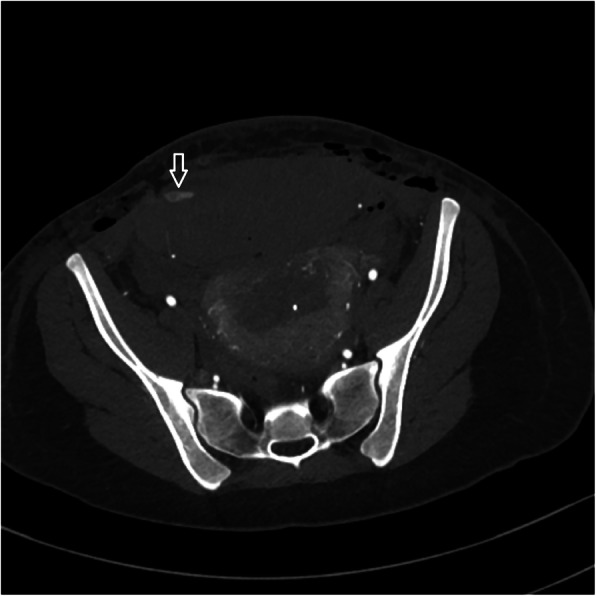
Case of a 16 year old patient status post cesarean section with decreasing hematocrit and hypotension. No signs of vaginal bleeding on exam. CT with active extravasation in the region of the right inferior epigastric artery with large rectus hematoma (arrow)
